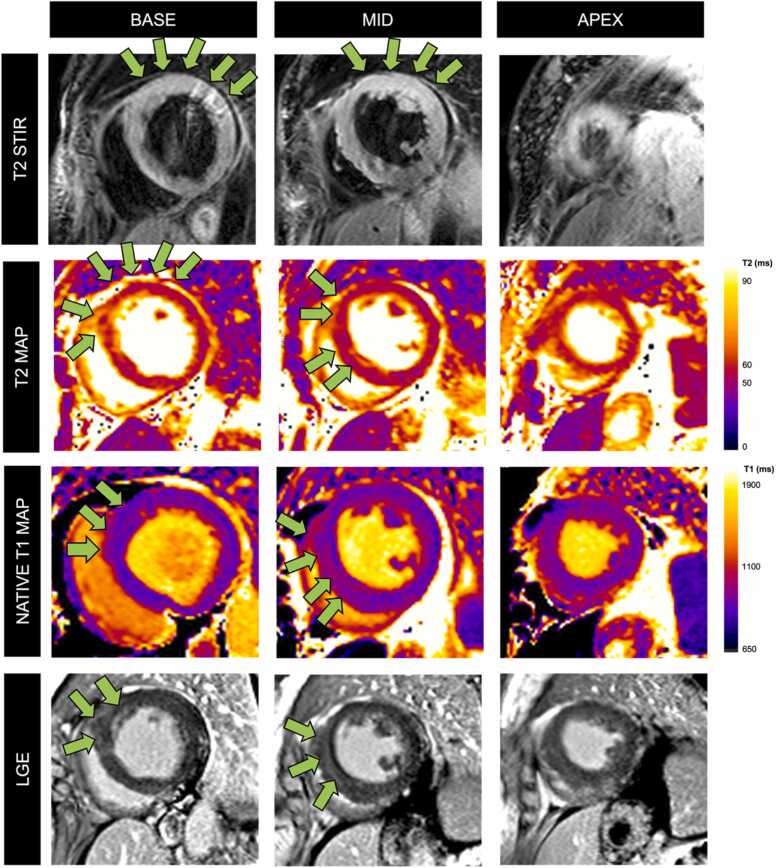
Myocarditis due to active systemic lupus erythematosus (SLE). A 62-year-old female with systemic lupus erythematosus presented with chest pain and breathlessness, associated with raised troponin I level of 177 ng/L (normal range <35 ng/L) and decreased C4 levels of <0.08 g/L (normal range 0.15–0.57 g/L) and raised ESR levels of 97 mm/h (normal range 0–12 mm/h). Transthoracic echocardiography showed moderately impaired LV systolic function with a LV EF of 43%. She was initiated on IV methylprednisolone and immunoglobulin due to highly suspected autoimmune myocarditis secondary to SLE. She subsequently underwent CMR which confirmed lupus myocarditis. T2W-STIR sequences revealed increased myocardial signal intensity. Parametric mapping reveals patchy increased areas of T2 values of 55–59ms (normal range <55 ms) and native T1 values of 1100 ms (normal range 890–1035 ms). LGE images show diffuse mid-wall enhancement. Myocardial injury is depicted by green arrows. C4= complement component 4; *CMR* cardiovascular magnetic resonance, *EF* ejection fraction, *ESR* erythrocyte sedimentation rate, IV intravenous, *LGE* late gadolinium enhancement, *LV* left ventricular, *T2-STIR* T2-short tau Inversion Recovery
